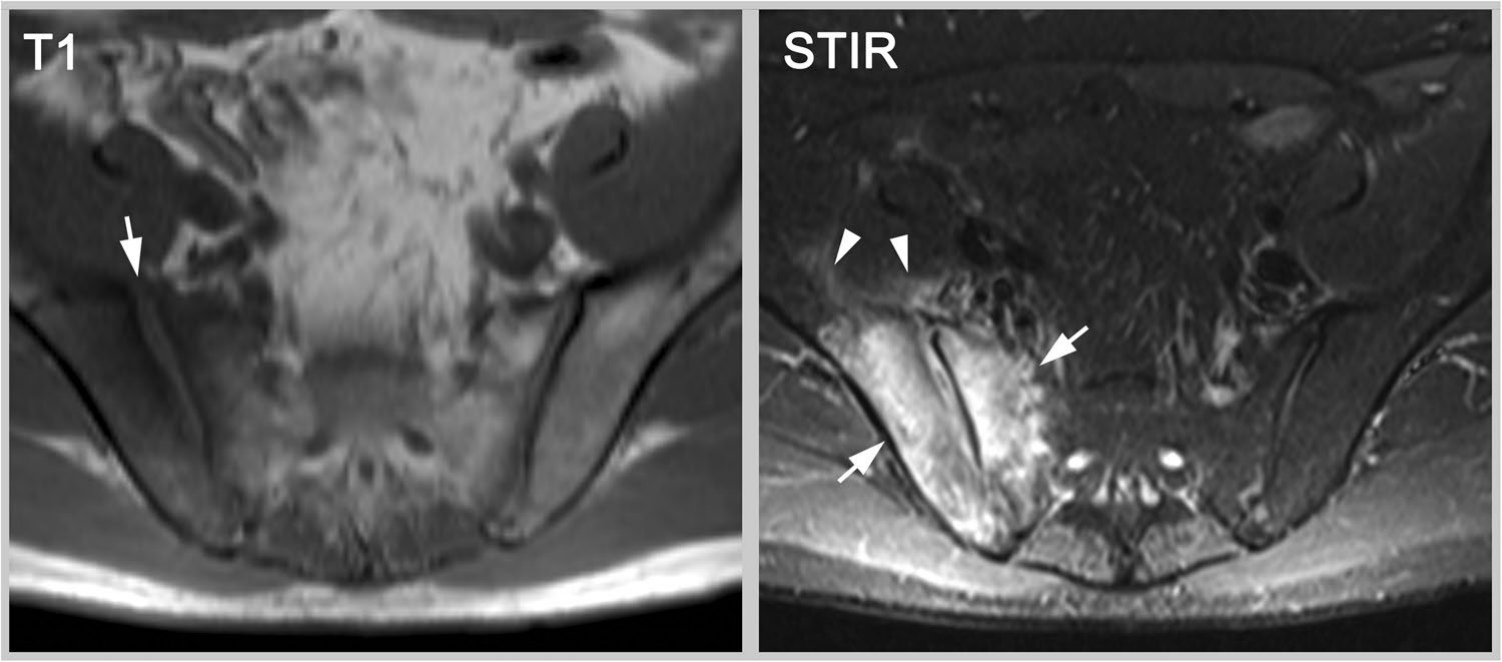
Septic sacroiliitis. MRI-T1 shows widening of the joint space from erosion (arrow) and MRI-STIR depicts severe bone marrow edema (arrows) and soft-tissue reaction (arrowheads). The extent of bone marrow edema is uncommon and the extent of soft-tissue inflammation is rare in axial spondyloarthritis, plus other structural lesions, such as fat metaplasia or backfill, are absent. Notably, there is also no lesion in the left SIJ
